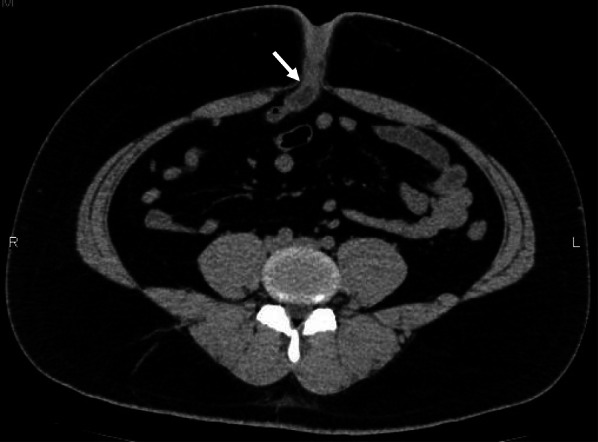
CT image at first visit. A luminal structure with liquid retention is evident just below the umbilicus (arrow)
